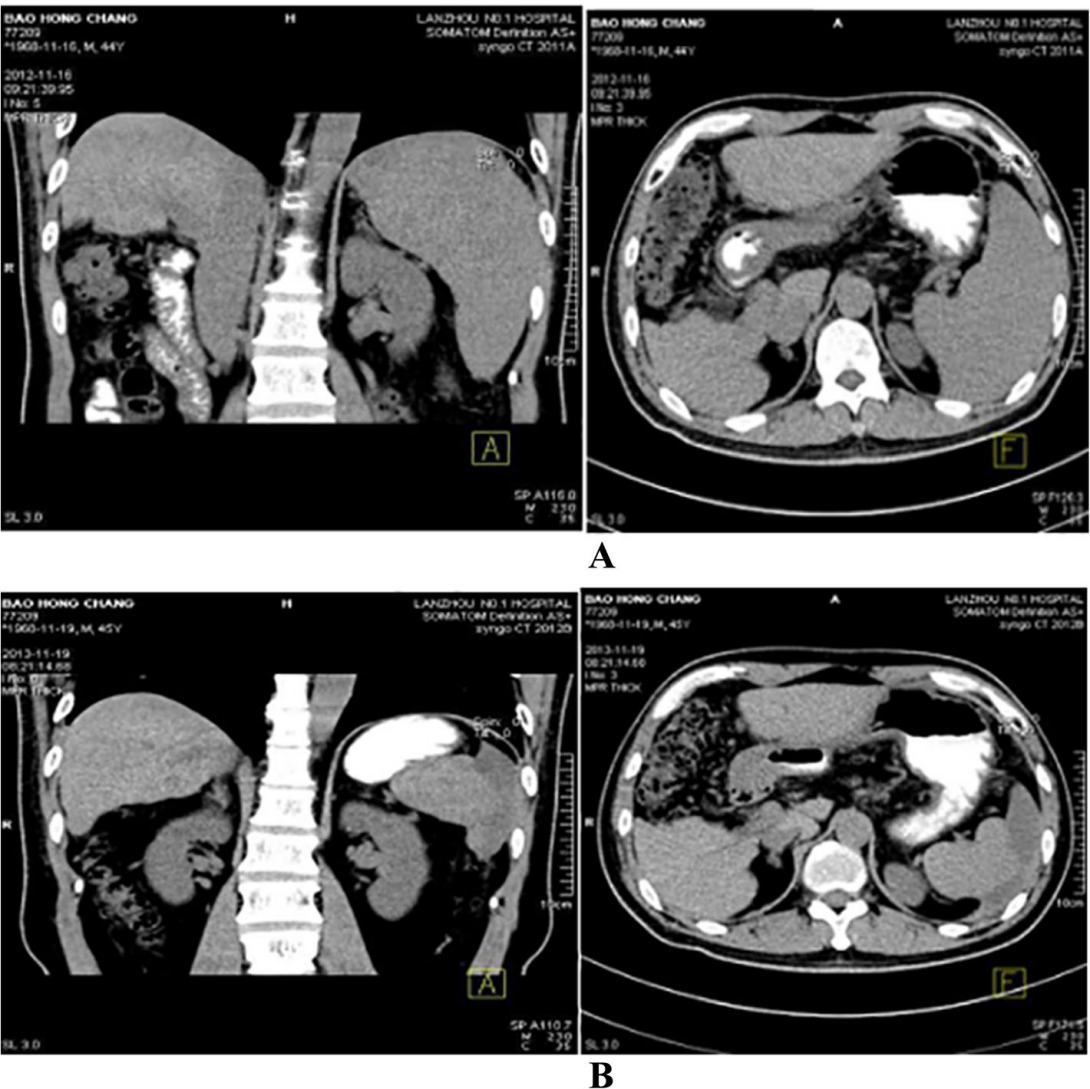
Comparison of upper abdominal CT before PSE and at 48 weeks after PSE. a. Upper abdominal non-enhanced CT before PSE and at 1 week after PSE in 2012. b. Upper abdominal non-enhanced CT at one year after PSE in 2013
